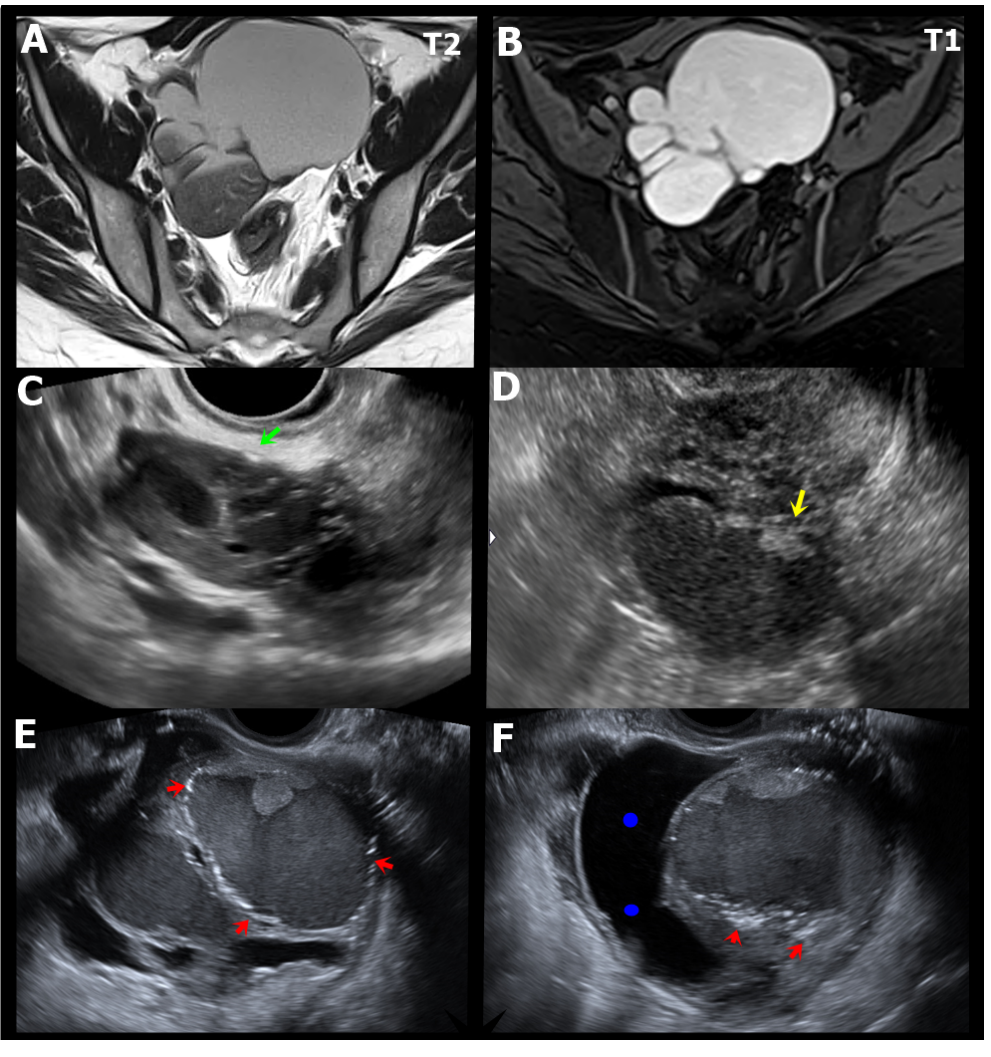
MRI and ultrasound appearances of ovarian endometriomas. A. Axial T2W MR image shows a multiloculated endometrioma with variable appearances of “T2 shading”. B. Axial T1W MR image with fat saturation shows a multiloculated endometrioma with marked and uniform hyperintensity with a “light bulb bright” appearance. C. Transvaginal ultrasound (TVUS) shows a small endometrioma with small echogenic focus in the ovarian parenchyma (green arrow).D. TVUS) shows an ovarian endometrioma with heterogeneous content due to small papillary projection (yellow arrow). E. TVUS shows two ovarian endometriomas, the largest with irregular contours and hyperechogenic focus in the wall (red arrows).F. TVUS showing an ovarian endometrioma with irregular contours and hyperechogenic focus in the wall (red arrows) associated with paraovarian inclusion cyst seen as an indirect sign of adherence process (blue dots).
